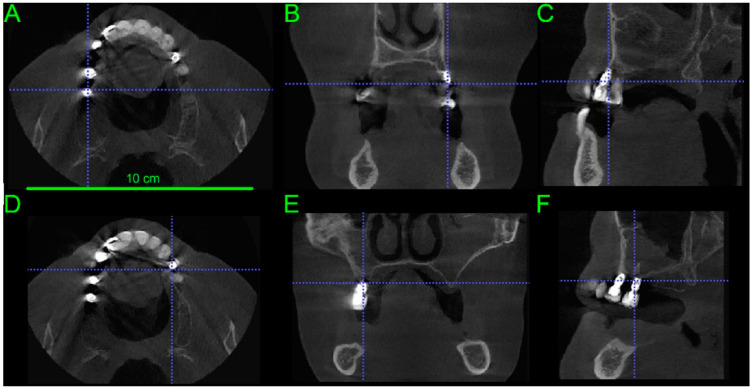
Bone quality determined pre-operatively using CBCT images. Orthogonal views were acquired by using 2D basis images for secondary reconstruction of axial (A,D), coronal (B,E) and sagittal (C,F) views. Significant buccal bone loss is apparent on the left side (upper panel), and the right side (lower panel).
